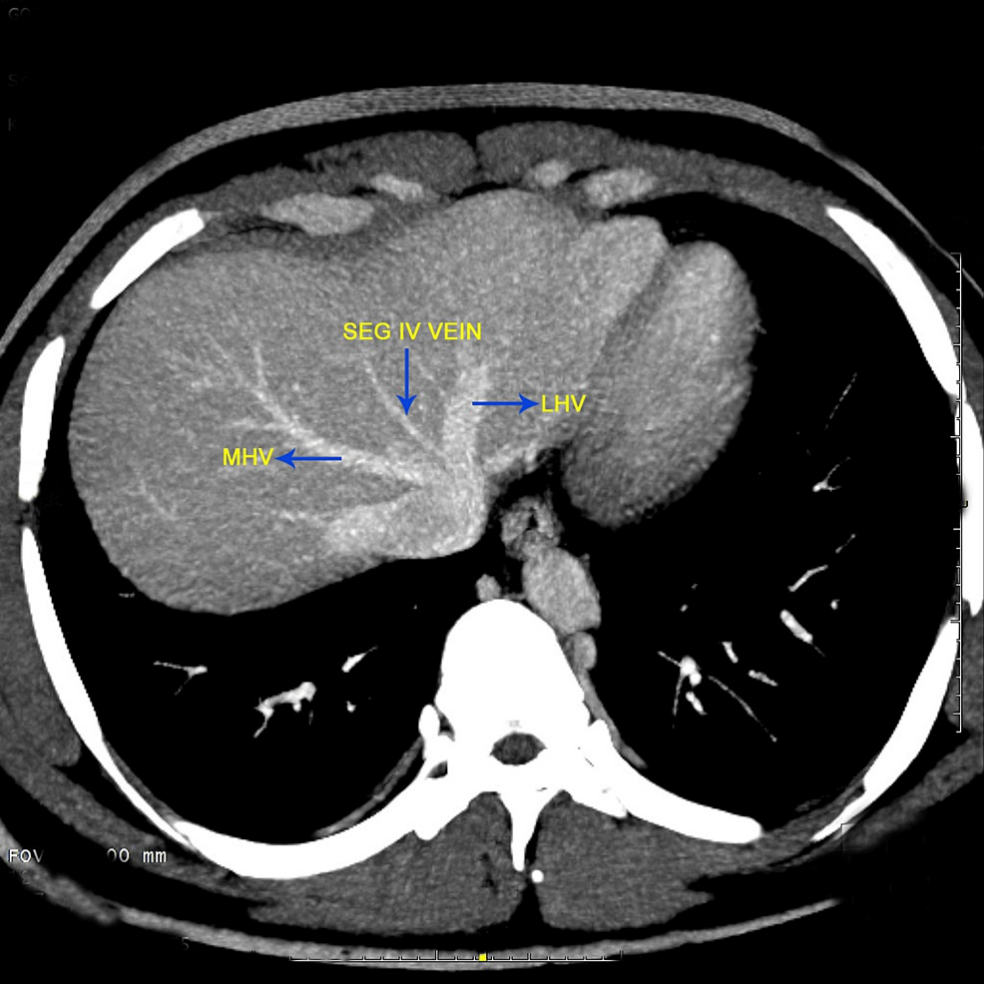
MDCT axial oblique view showing the segment IV drainage predominantly into the left hepatic vein (type I) MDCT: Multidetector computed tomography (The figures are from the patients included in the current study.)
